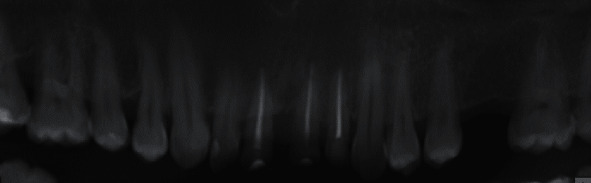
No pathology and resorption. 3-year radiograph examination.
